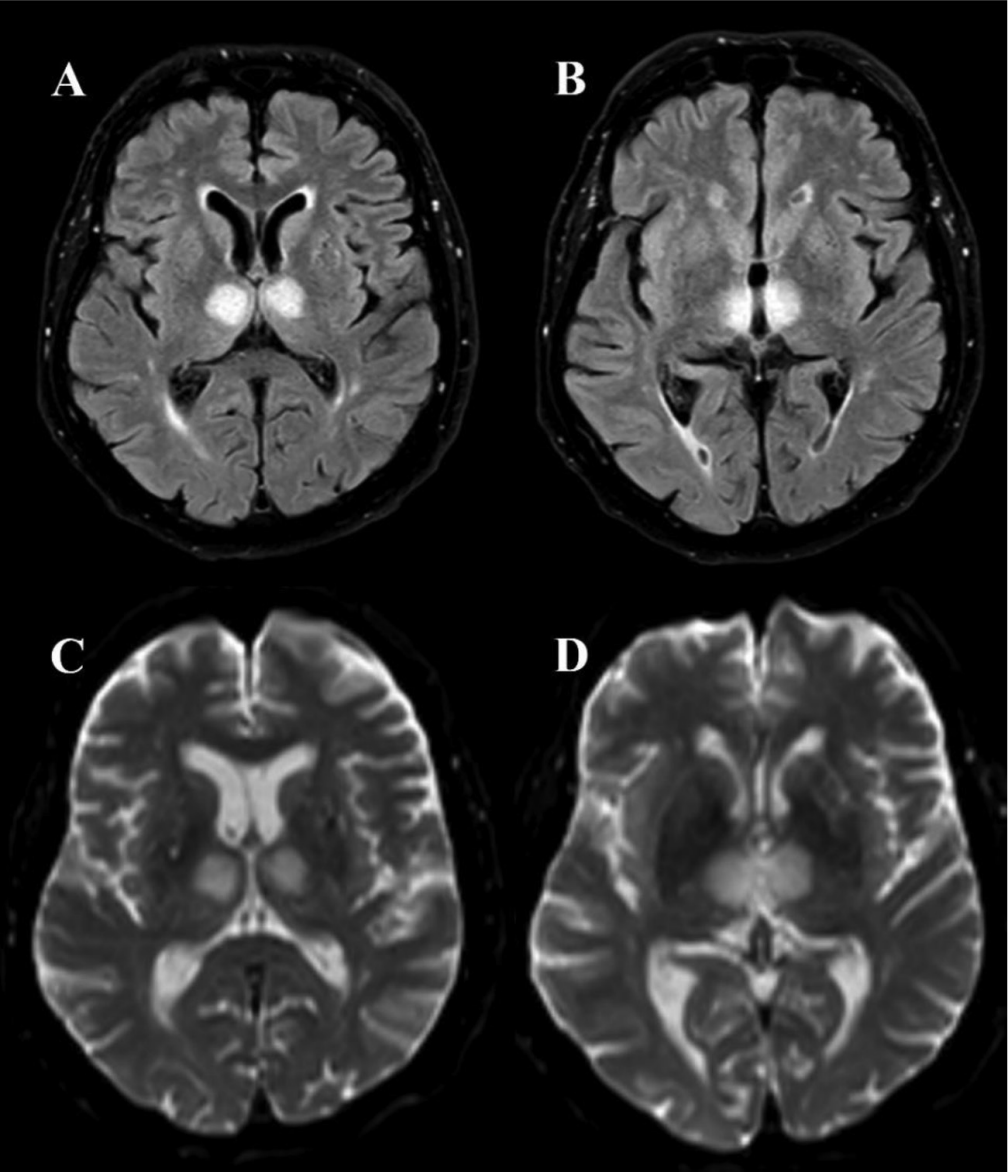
Axial MRI images: FLAIR (A and B) and DWI (C and D) sequences, showing hyperintense bilateral paramedian thalamic signal. MRI, magnetic resonance imaging; FLAIR, fluid attenuated inversion recovery; DWI, diffusion weighted imaging
